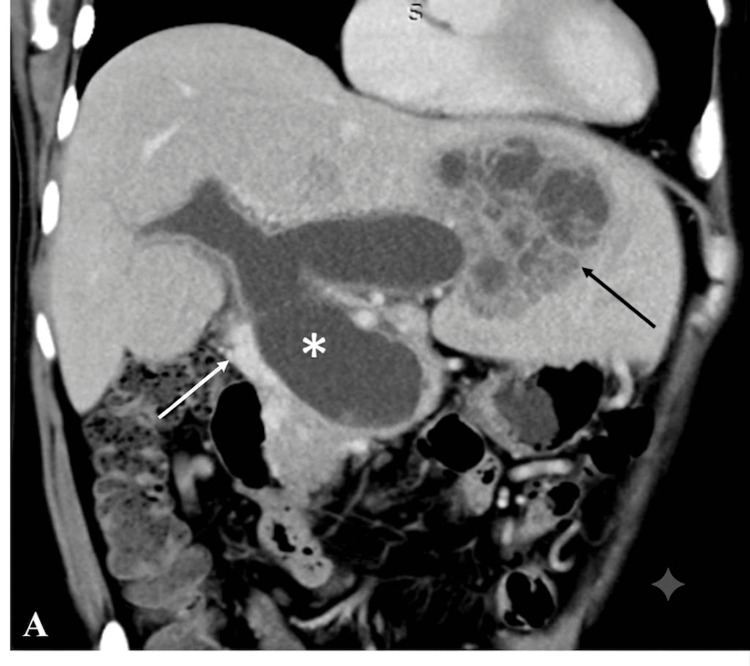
Contrast-enhanced CT demonstrating a pancreatico-portal fistula with portal vein distension and cavernous transformation. The white asterisk indicates cystic contents within the distended portal vein; the thin black arrow indicates a multiseptated cystic lesion in the left hepatic lobe; the thin white arrow indicates a portal cavernoma.
